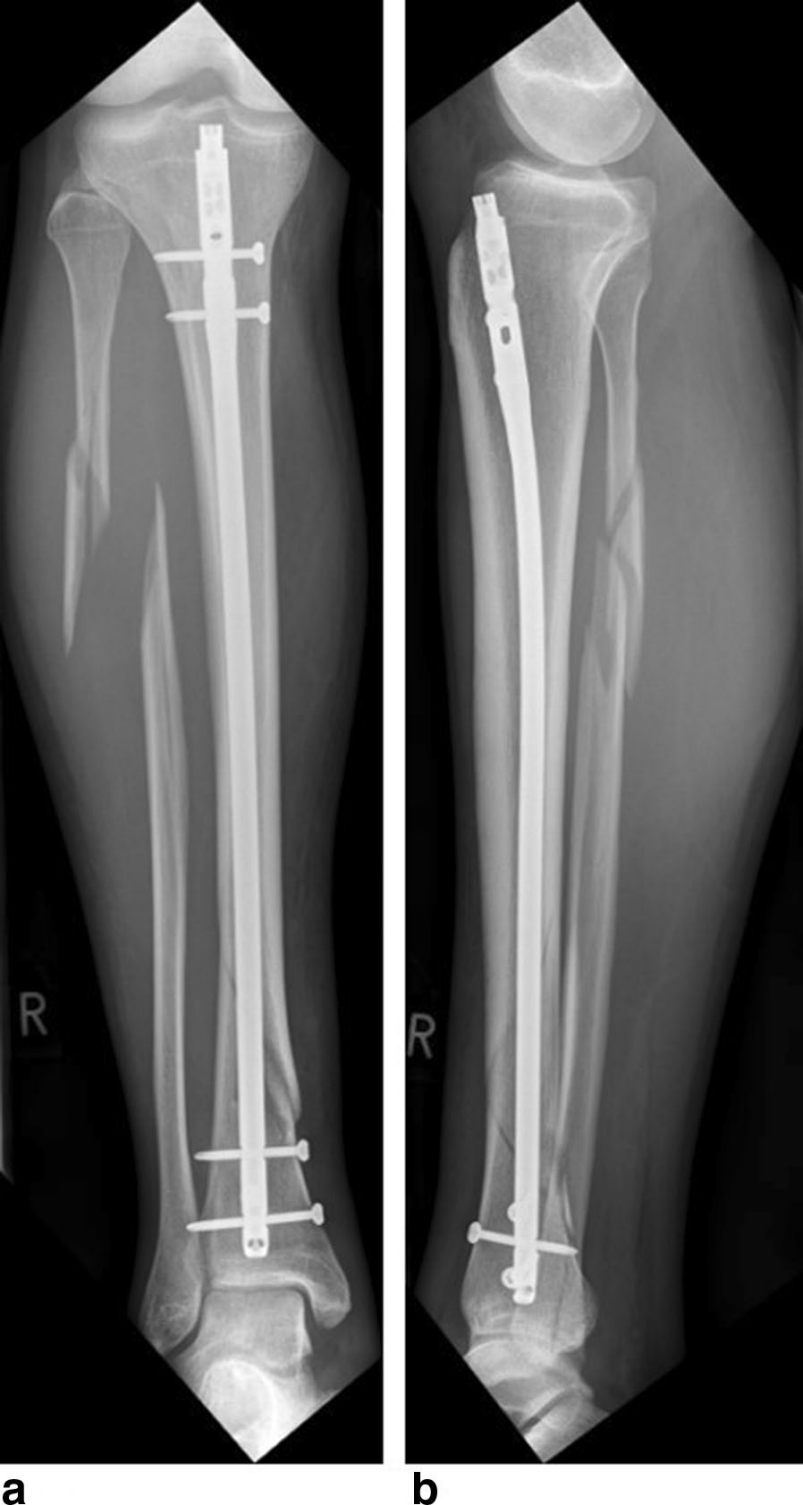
Anterio-posterior (a) and medio-lateral (b) radiographs of the right lower leg showing fracture reduction
